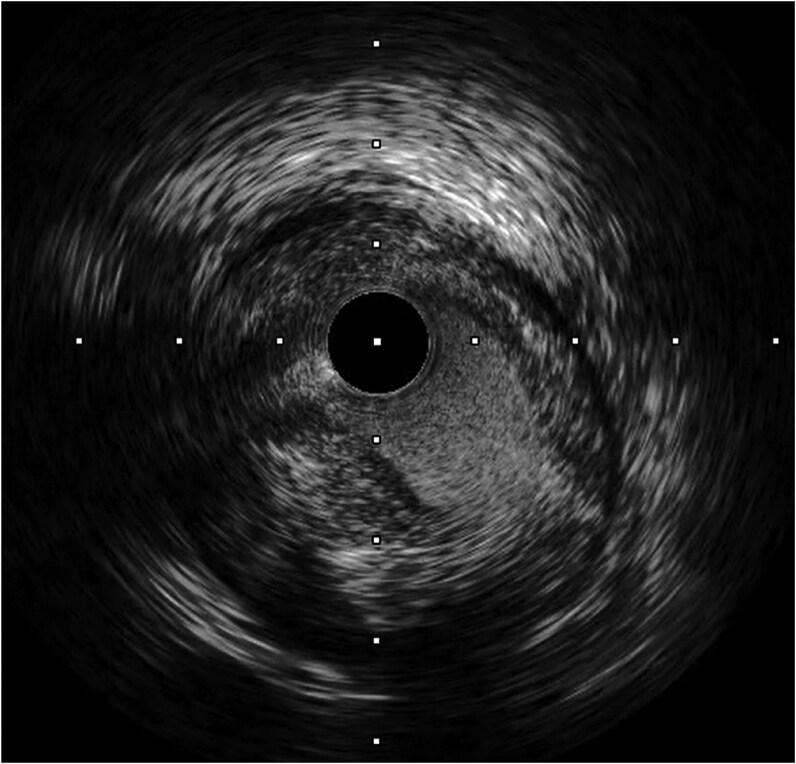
Intravascular ultrasound findings of proximal right coronary artery. Intravascular ultrasound demonstrated a mixed plaque with both fibrous and lipid components and the presence of thrombus, suggesting a relatively acute lesion responsible for sinus node artery ischaemia (Supplementary material online, *Video 3*).
